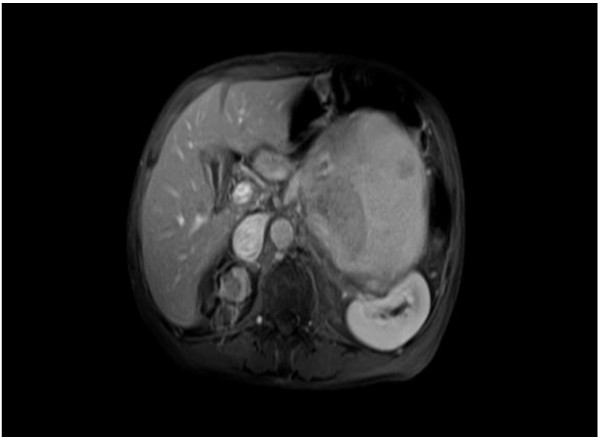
Abdominal magnetic resonance imaging (MRI). The T1-weighted images show high signal intensity and many partition walls.
